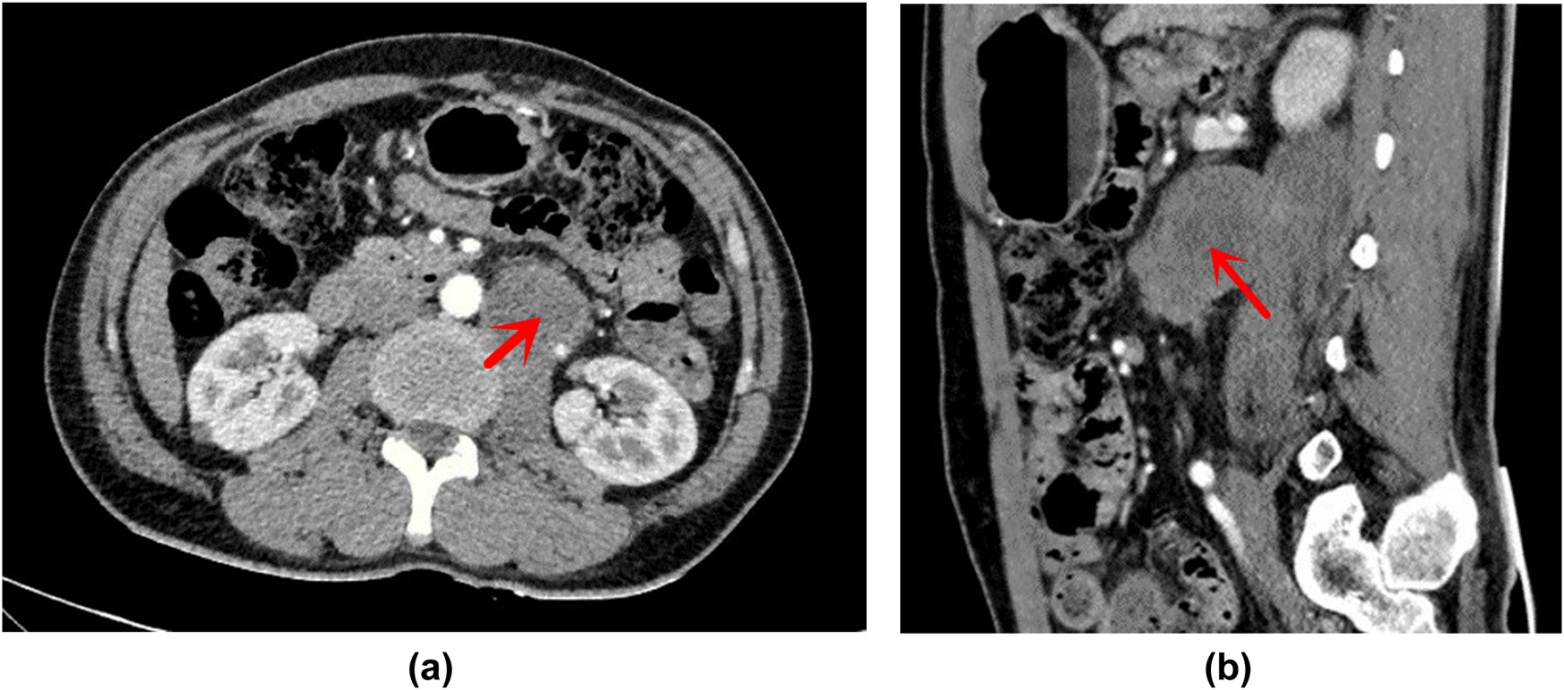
(a) Computed tomography (CT) cross section: low-density area of soft tissue mass in the front edge of the left retroperitoneal psoas major muscle. (b) The CT sagittal plane: low-density area of soft tissue mass in the front edge of the left retroperitoneal psoas major muscle.
